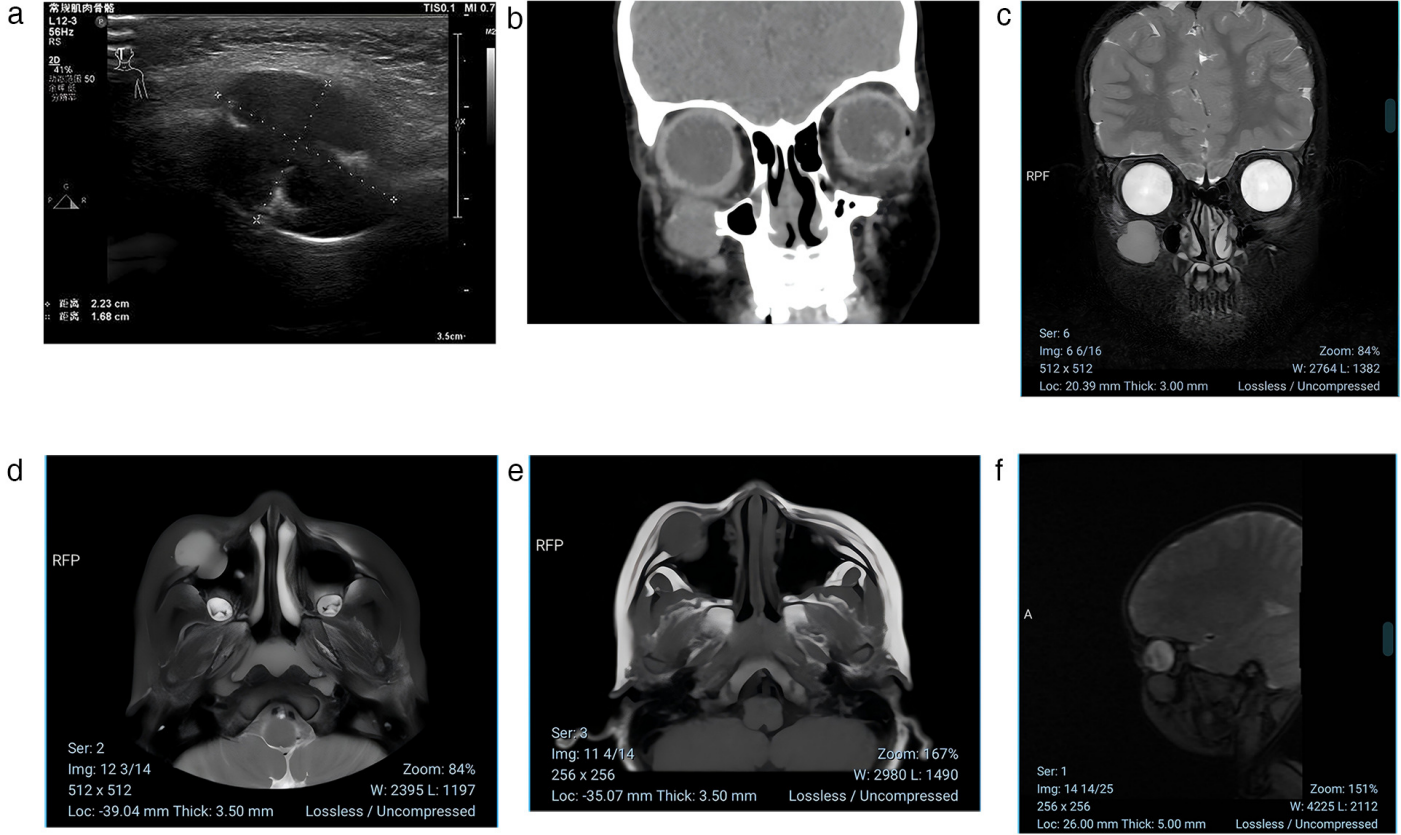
(a) Ultrasound examination indicated a mass in the anterior wall of the right maxillary sinus. The lesion measures approximately 2.23 cm × 1.68 cm in maximum diameter, demonstrating clear boundaries and heterogeneous echogenicity. (b) A coronal CT scan of the paranasal sinuses revealed a mass in the area of the right maxillary sinus anterior wall. Coronal CT scan showing an oval-shaped isodense lesion in the region of the right maxillary sinus anterior wall and infraorbital canal. The lesion measures approximately 1.5 cm × 2.1 cm, with well-defined margins and uniform density (CT value approximately 30–35 HU). Notable expansion is observed, with protrusion into both the maxillary sinus cavity and subcutaneous tissue, accompanied by localized bone destruction of the anterior wall of the maxillary sinus. (c–f) MRI scan showing an oval-shaped abnormal signal in the region of the right maxillary sinus anterior wall and infraorbital canal. On T2-weighted imaging (T2WI) and FLAIR sequences, the lesion appears hyperintense, while it is hypointense on T1-weighted imaging (T1WI). The lesion measures approximately 1.5 cm × 2.1 cm and exhibits significant heterogeneous enhancement on post-contrast scans.
